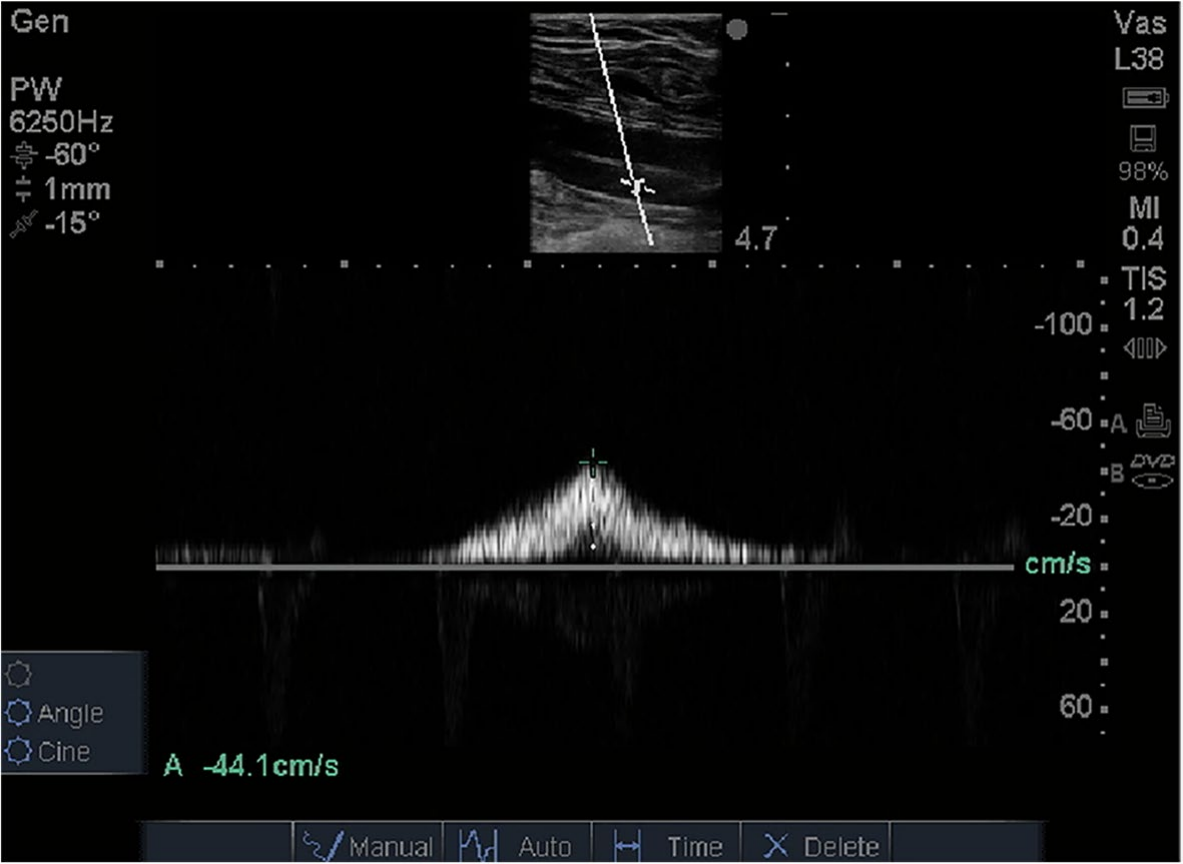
The Doppler cursor was placed in the centre of the vein and peak blood velocity were measured
